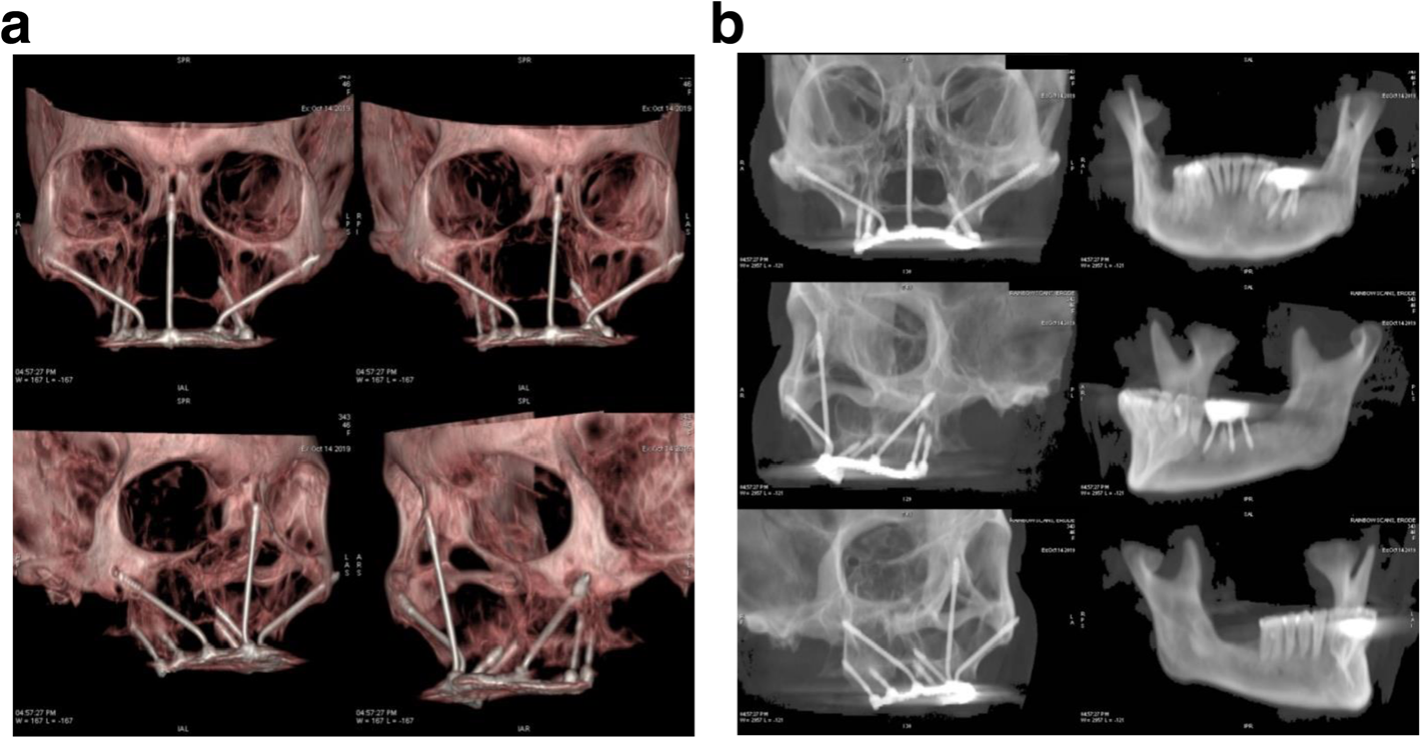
a Post-operative CT coronal view: one zygomatic implant was used for the glabella region anchorage, engaging the floor of the frontal sinus, and in the mandible, three implants were placed in order to replace the left molar. b Post-operative CT sagittal view
